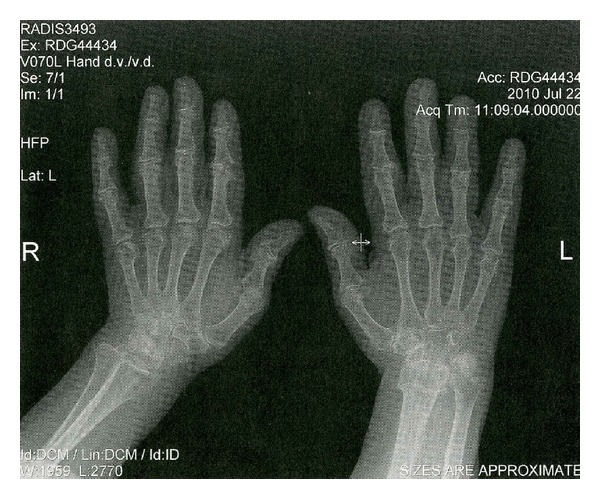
Radiological changes in acromegalic arthropathy on X-ray scan (with permission of Peter Vaňuga, MD, PhD., National institute of endocrinology and diabetology, L'ubochňa, Slovakia).
